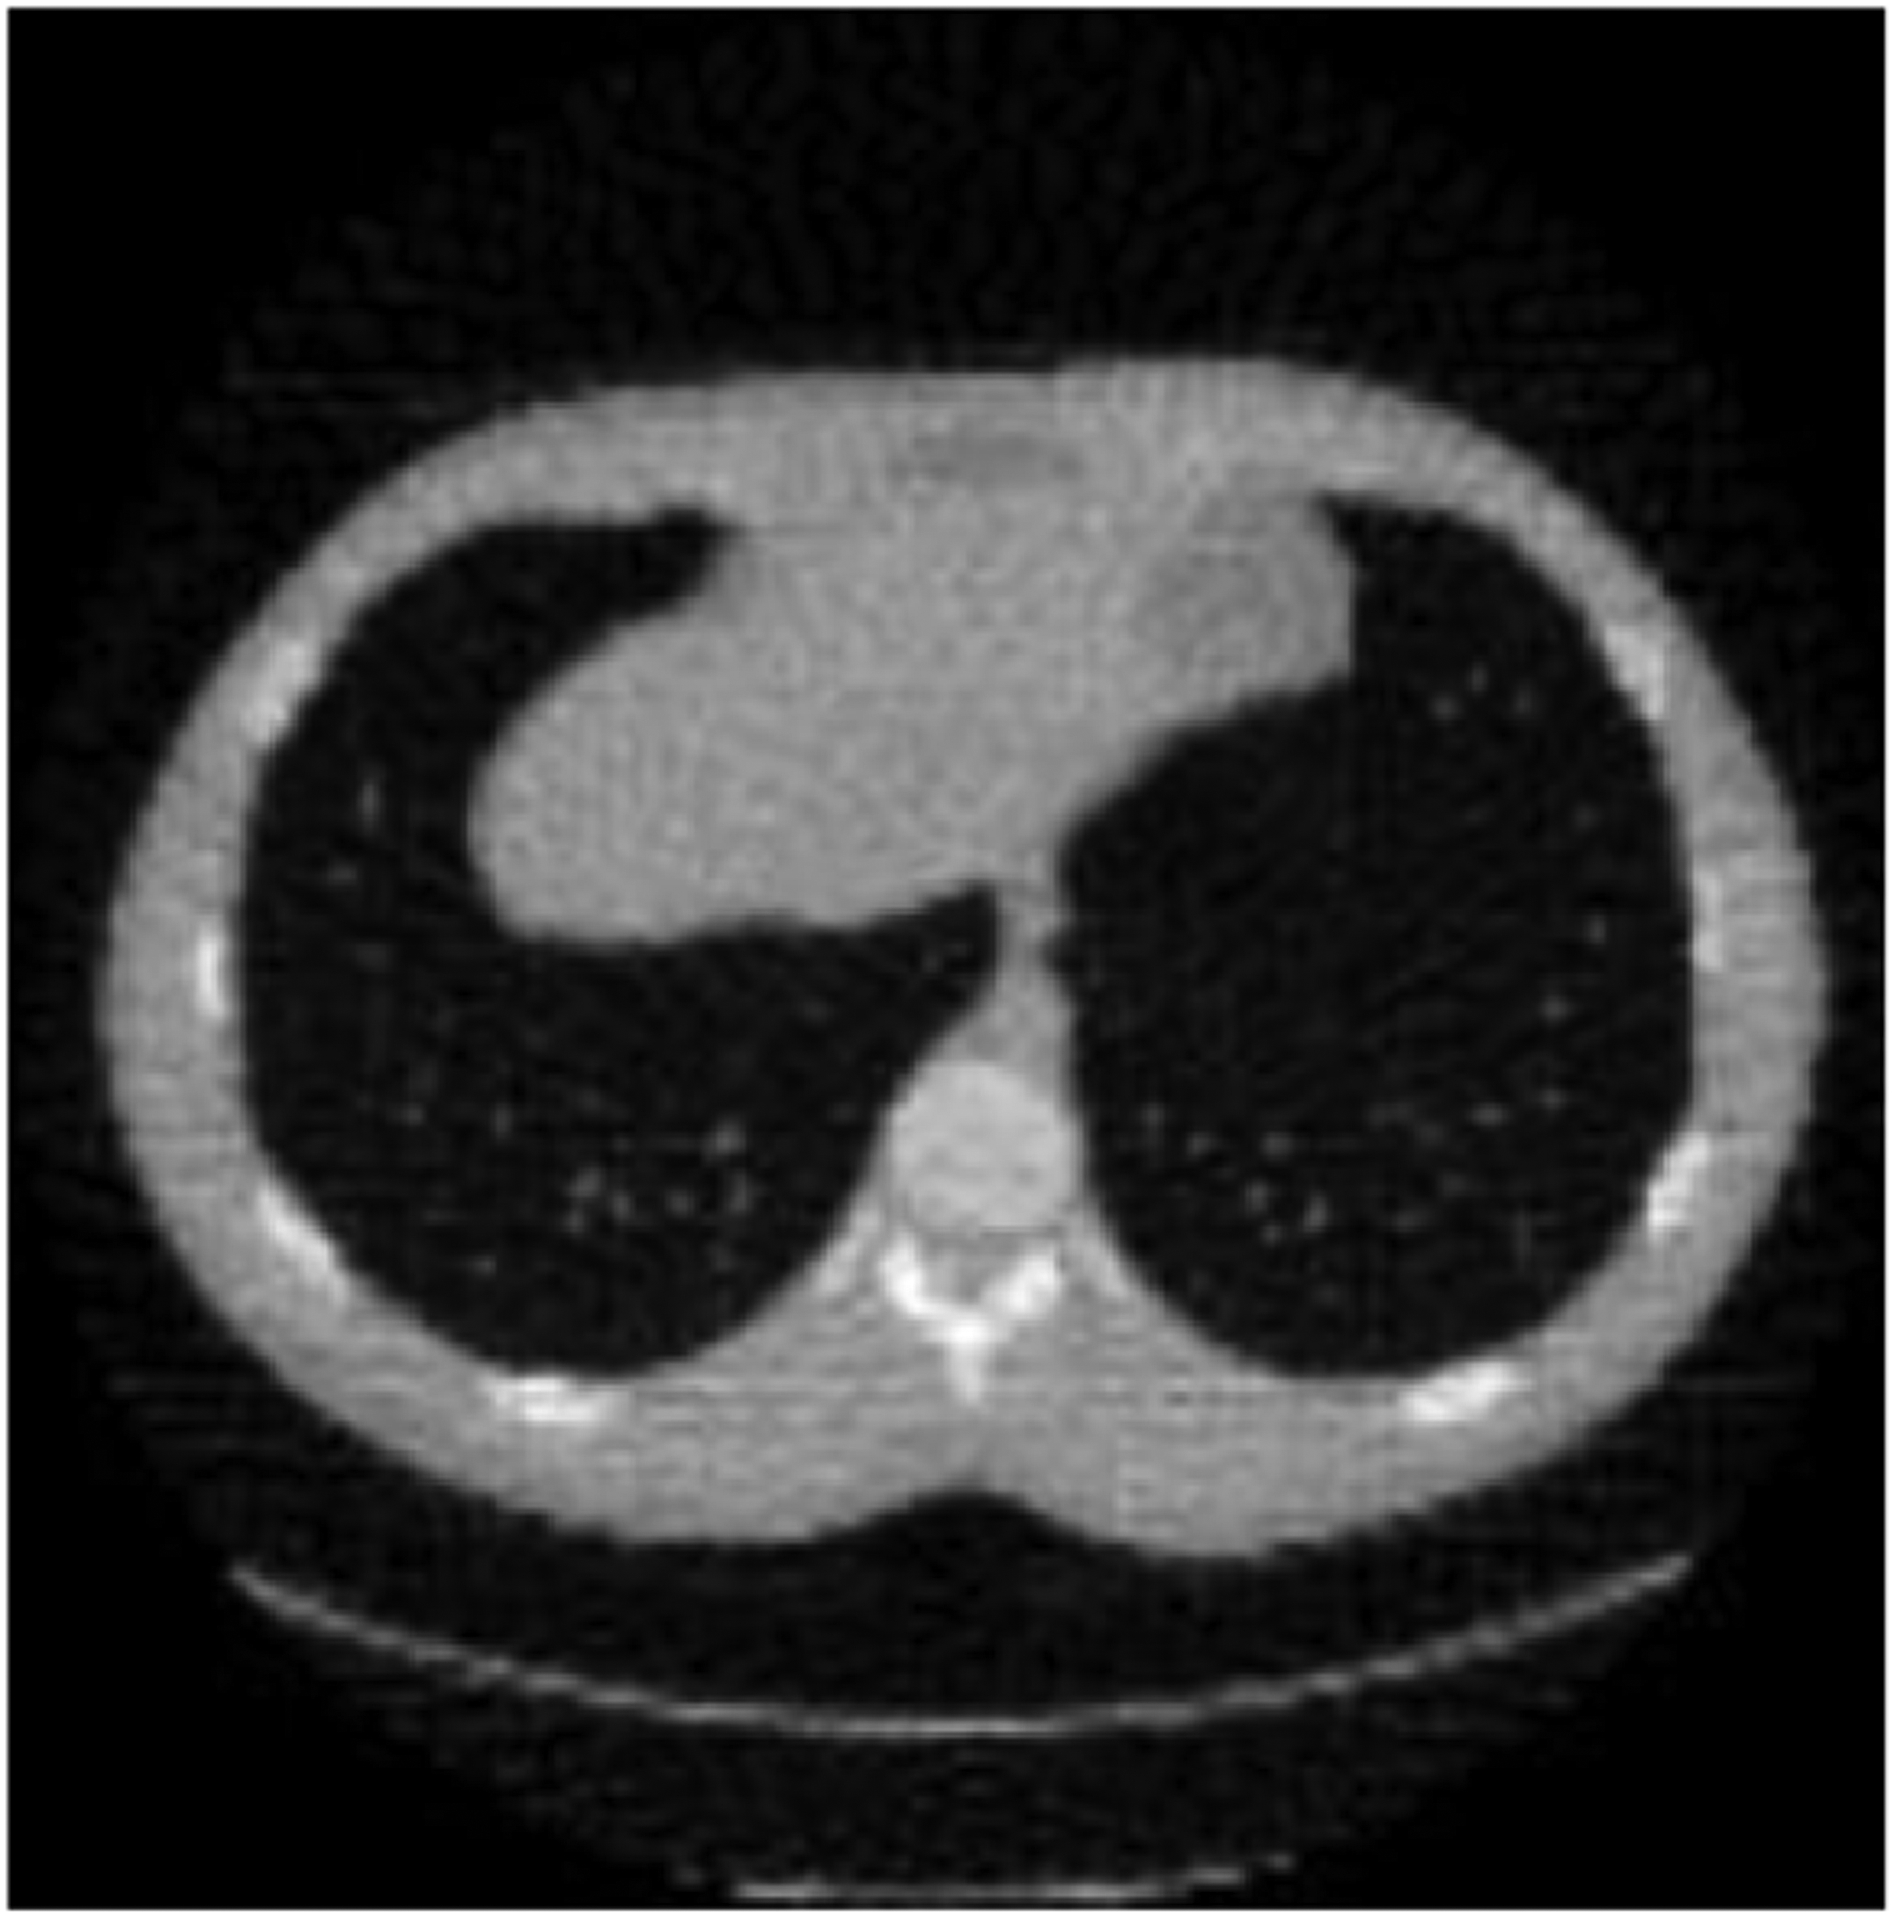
Reconstruction from full sonogram.
